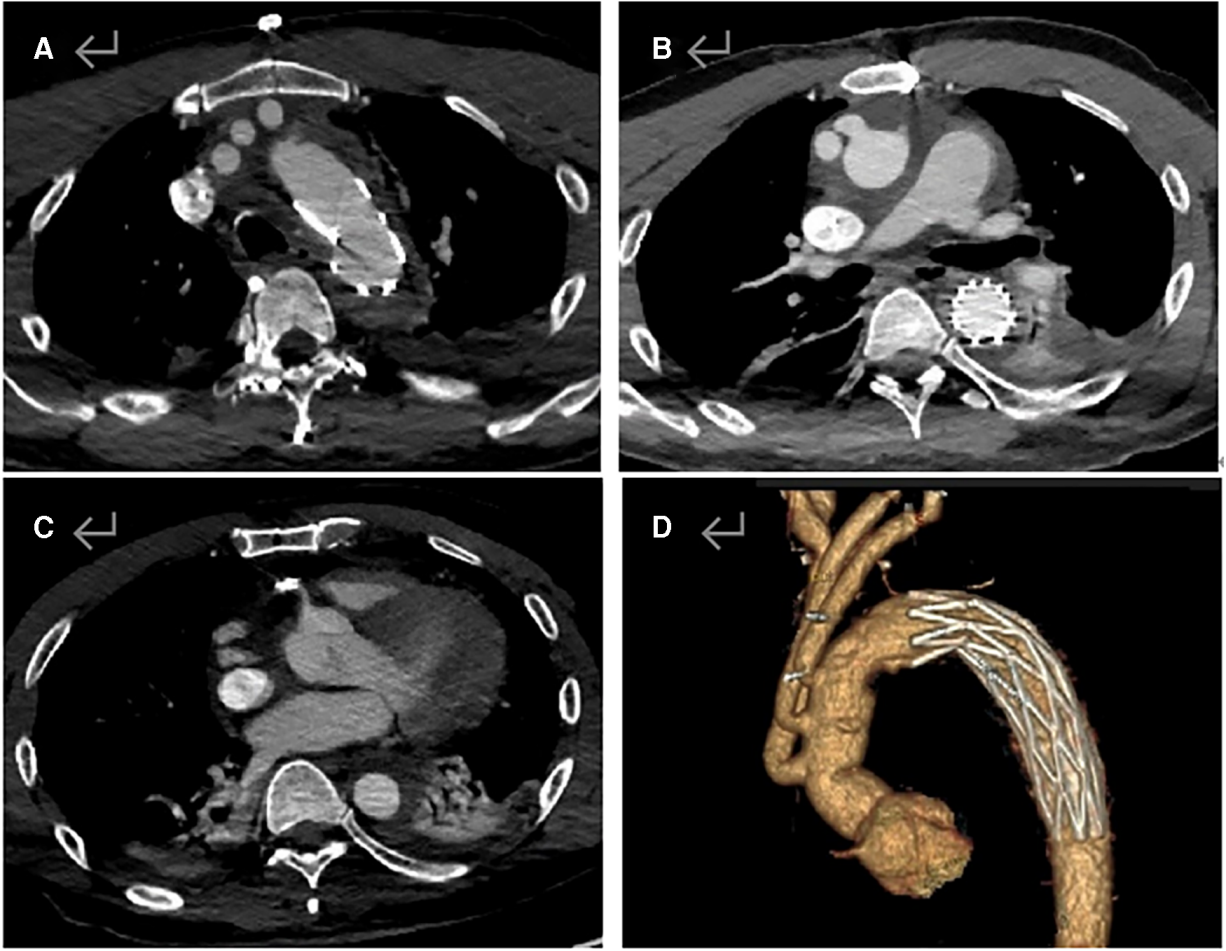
Postoperative aortic CTA image. (A) Artificial vessels in the aortic arch and the cephalobrachial artery are unobstructed. (B) Ascending aorta is unobstructed, and the stent is well dilated without internal leakage. (C) Opening of the right coronary artery is unobstructed, and stent implantation is visible. (D) Smooth morphology of the thoracic aorta.
